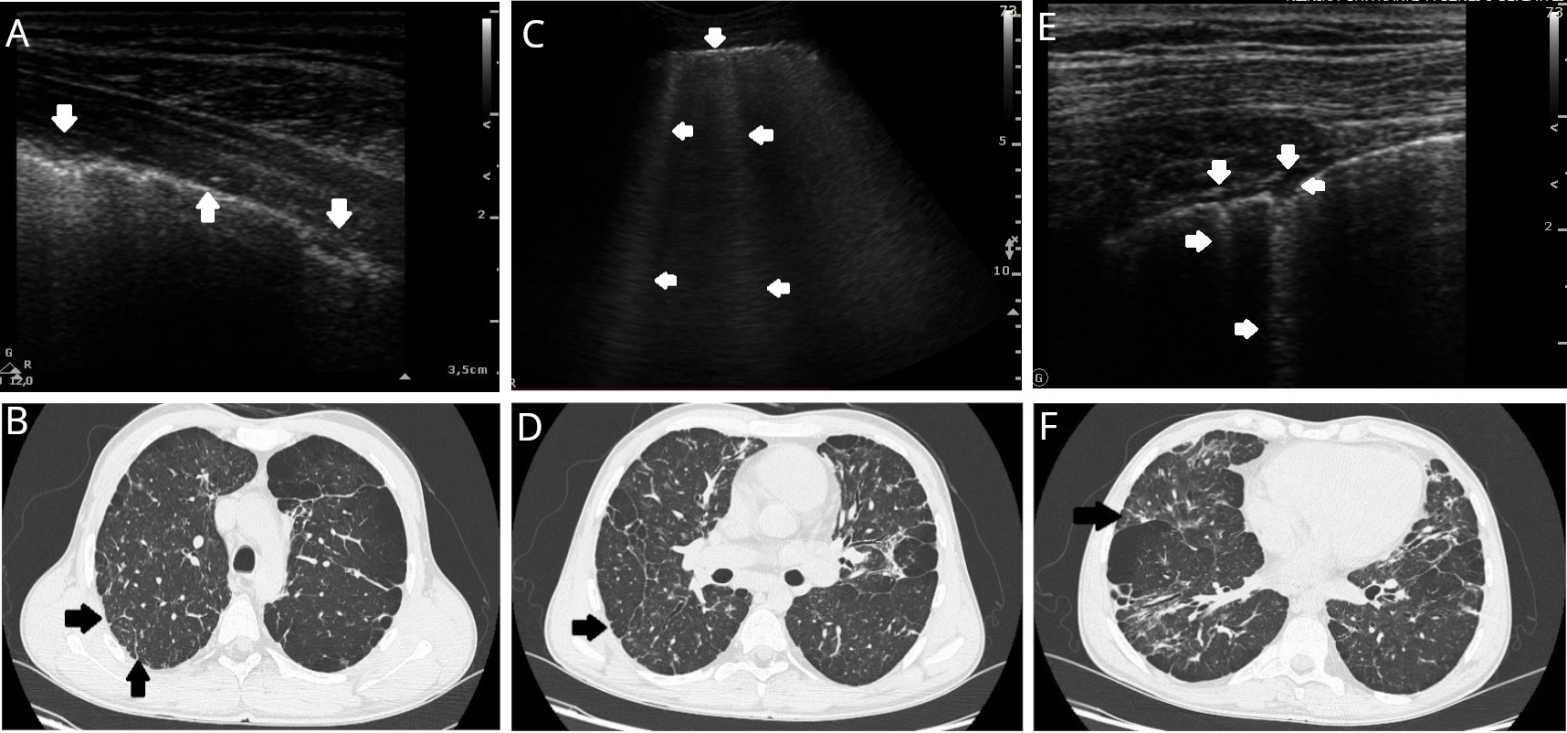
Examples of lung lesions in a patient with predominantly antibody deficiency on lung ultrasound (LUS) and high-resolution tomography (HRCT). (A) LUS: Irregular and infiltrated pleural line (↓), regular pleural line (↑). Linear probe. (B) HRCT (axial plane): Right lung - peripheral paraseptal emphysema with centrilobular emphysema inside the lung and fibrosis, thickening of the pleura between the arrows with subpleural fibrosis (arrowheads). Left lung combined centrilobular and panlobular emphysema with fibrosis. (C) LUS: Irregular pleural line (↓) and multiple B lines (←). Convex probe (1-6MHz). (D) HRCT (axial plane): Right lung - peripheral paraseptal emphysema with centrilobular emphysema inside the lung and fibrosis (arrowhead). Left lung combined paraseptal and centrilobular emphysema with fibrosis. (E) LUS: Irregular pleural line, and small subpleural consolidation (↓←) with vertical artifact C line (arising from subpleural lesion) (→). Linear probe. (F) HRCT (axial plane): Right lung – combined paraseptal, centrilobular and panlobular emphysema and fibrosis, thickening of the pleura with subpleural fibrosis (arrowhead). Left lung combined paraseptal, centrilobular and panlobular emphysema with fibrosis.
